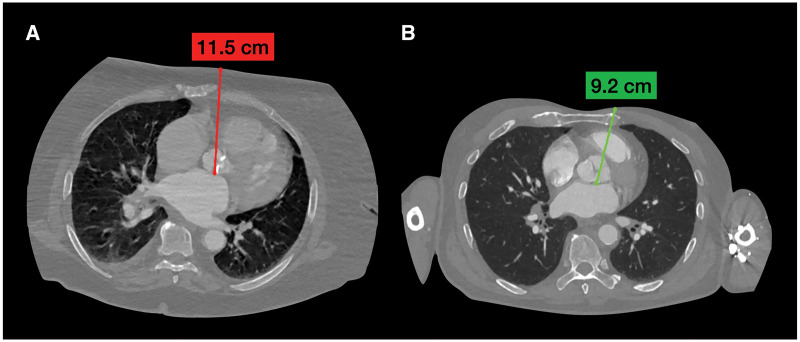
Computed tomography distance from the posterior aortic valve annulus to the skin level. (A) Knot-pusher was used during aortic valve replacement. (B) Knot-pusher was not used.
